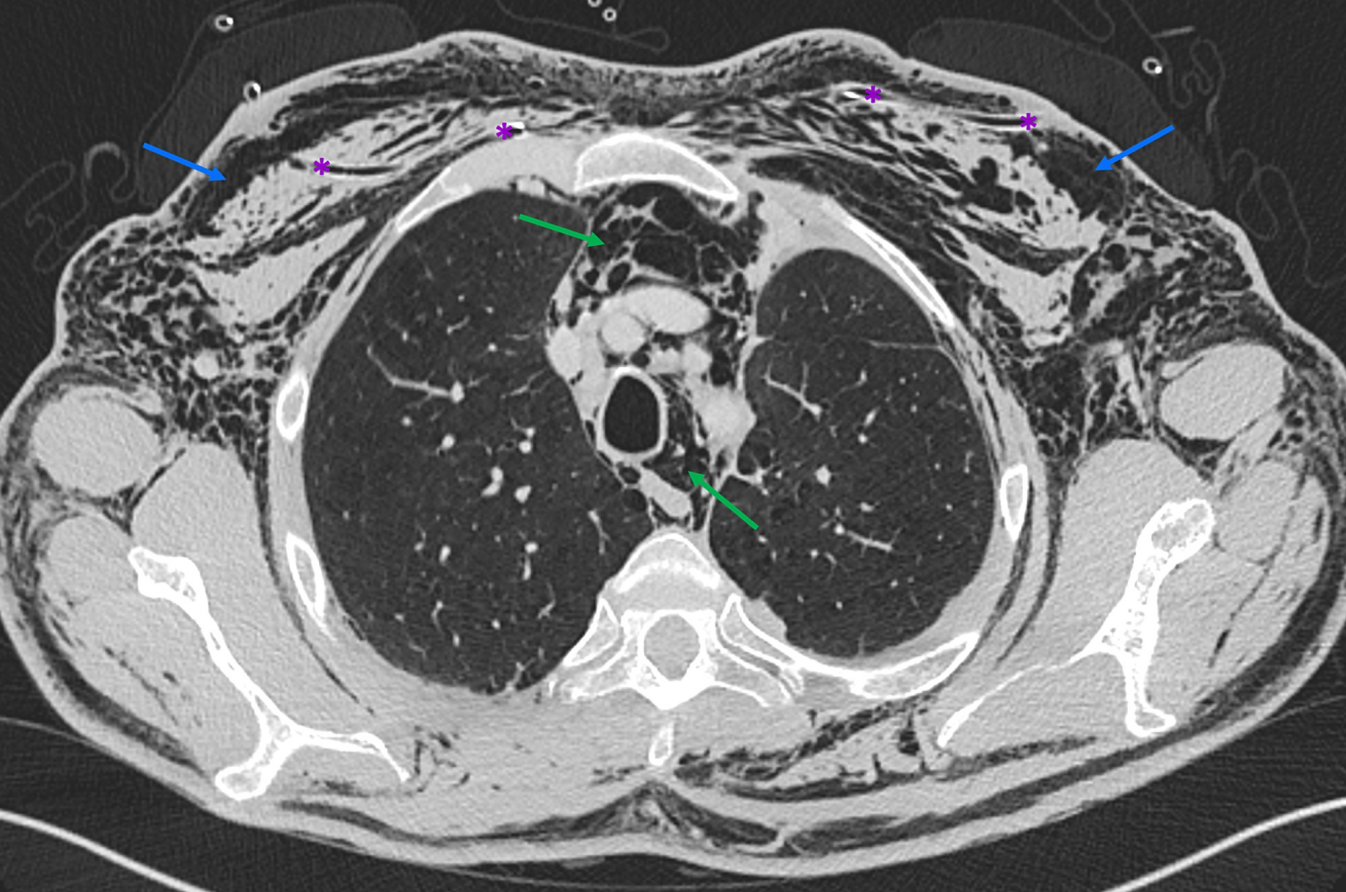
Non-contrast chest CT, lung window, and axial plane Non-contrast chest CT, lung window, and axial plane show slight reduction in the extent of pneumomediastinum (green arrows) and subcutaneous emphysema (blue arrows). Note bilateral subcutaneous tubes (purple asterisks).
